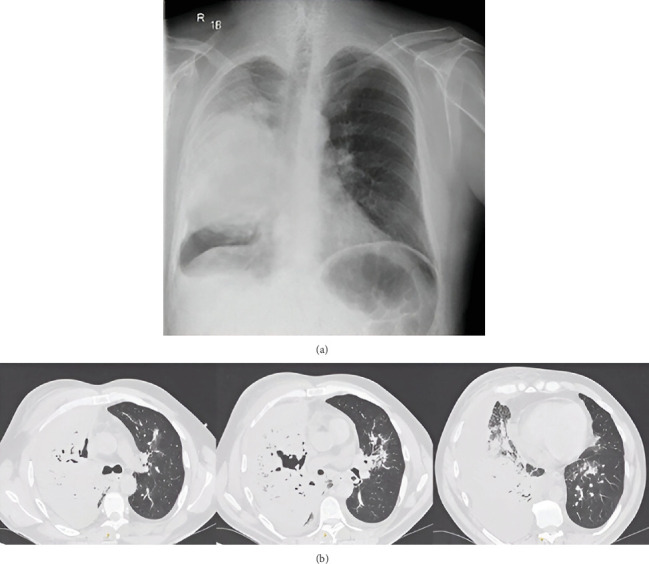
(a) Patient's chest x-ray performed on admission on the 13th of August 2022. (b) CT scans of the chest taken on the 17th of August 2022—Case 2.
